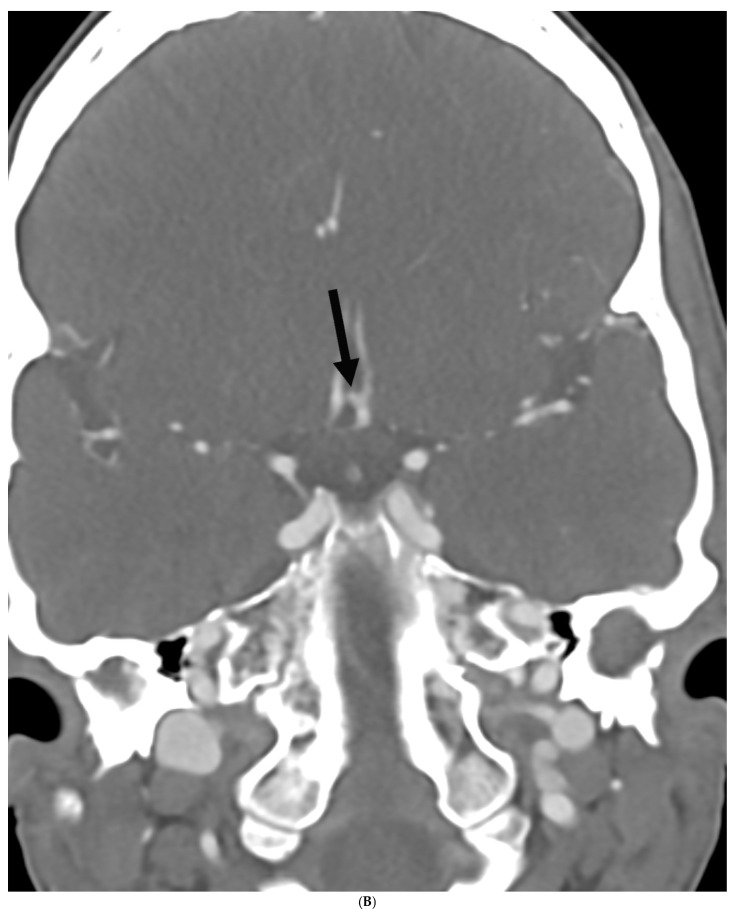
(A) 24-year-old woman with hEDS and a history of chronic migraine. Sagittal CT angiography image demonstrates a 2 mm saccular aneurysm from the left pericallosal artery (arrow). (B) 36-year-old woman with HSD and a history of chronic migraine. Coronal CT angiography image demonstrates a 2 mm left A1/A2 junction aneurysm (arrow).
